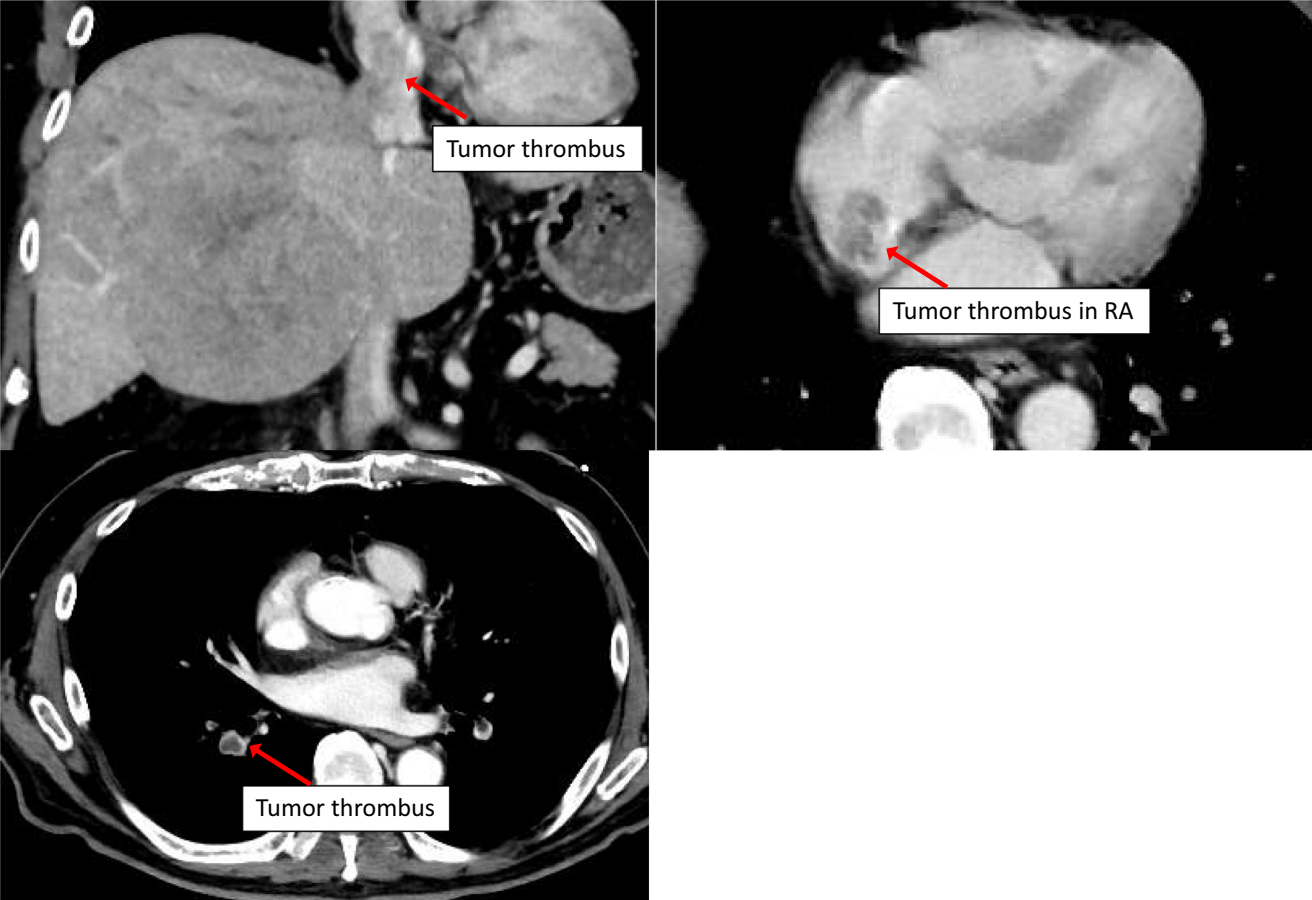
Abdominal computed tomography showed a pulmonary embolism and the tumor thrombus extension into the right atrium
